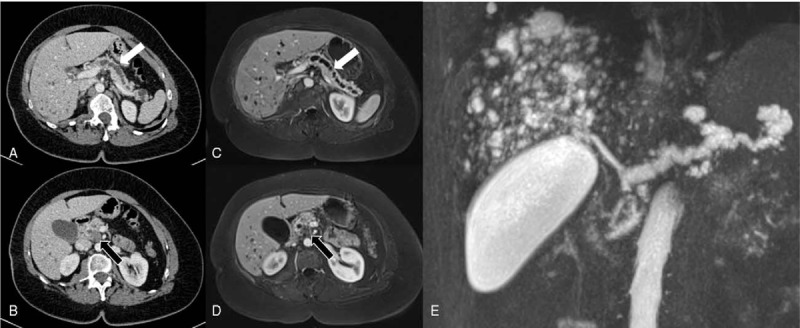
Cross sectional imaging. A: Portal phase enhanced CT scan showing main pancreatic duct dilatation (white arrow). B: Portal phase enhanced CT scan showing pancreatic head adenocarcinoma (black arrow). C: T1 Gadolinium enhanced MRI showing main pancreatic duct dilatation (white arrow). D: Gadolinium enhanced MRI showing pancreatic head adenocarcinoma (black arrow). E: CP-MRI showing main pancreatic duct dilatation.
